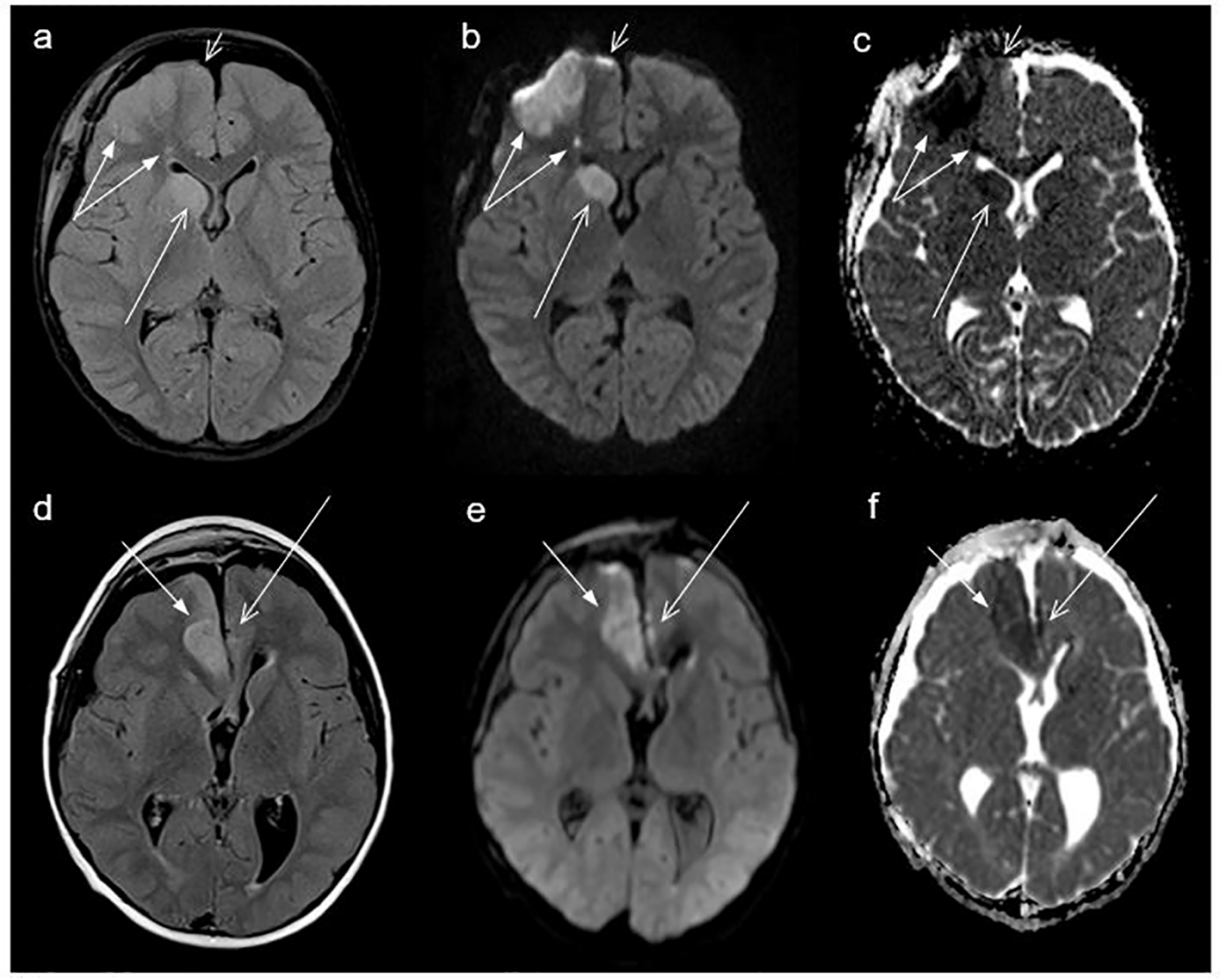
Cranial magnetic resonance imaging (MRI) of a craniopharyngioma patient (case #25, Table 2) with cerebral infarction (CI) of middle cerebral artery (MCA) on the right side (double arrow, territorial CI), anterior cerebral artery ACA (Heubner) right side (long arrow), and the top of ACA right side (short arrow, paired with contusions), performed 4 days after surgery (A–C); and MRI of a craniopharyngioma patient (case #19, Table 2) with cerebral infarction (CI) of the anterior cerebral artery (ACA) on the right side (short arrow) and on the left side (long arrow) showing a linear cortical CI, MRI performed one day after surgery (D–F). (A, D) show fluid-attenuated inversion recovery (FLAIR) sequences, (B, E) show DWI b1000, and (C, F) show apparent diffusion coefficient (ADC) images, all axial plane.
